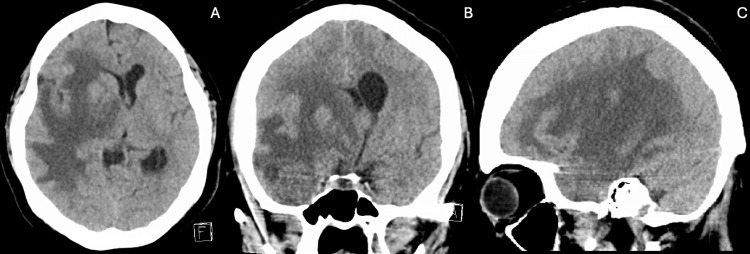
Right sphenoid wing meningioma observed on non-contrast brain CT scan. Non-contrast brain CT in axial (A), coronal (B), and sagittal (C) views demonstrates a lesion located at the right temporal pole with significant perilesional vasogenic edema, causing a severe midline shift of over 1 cm.
